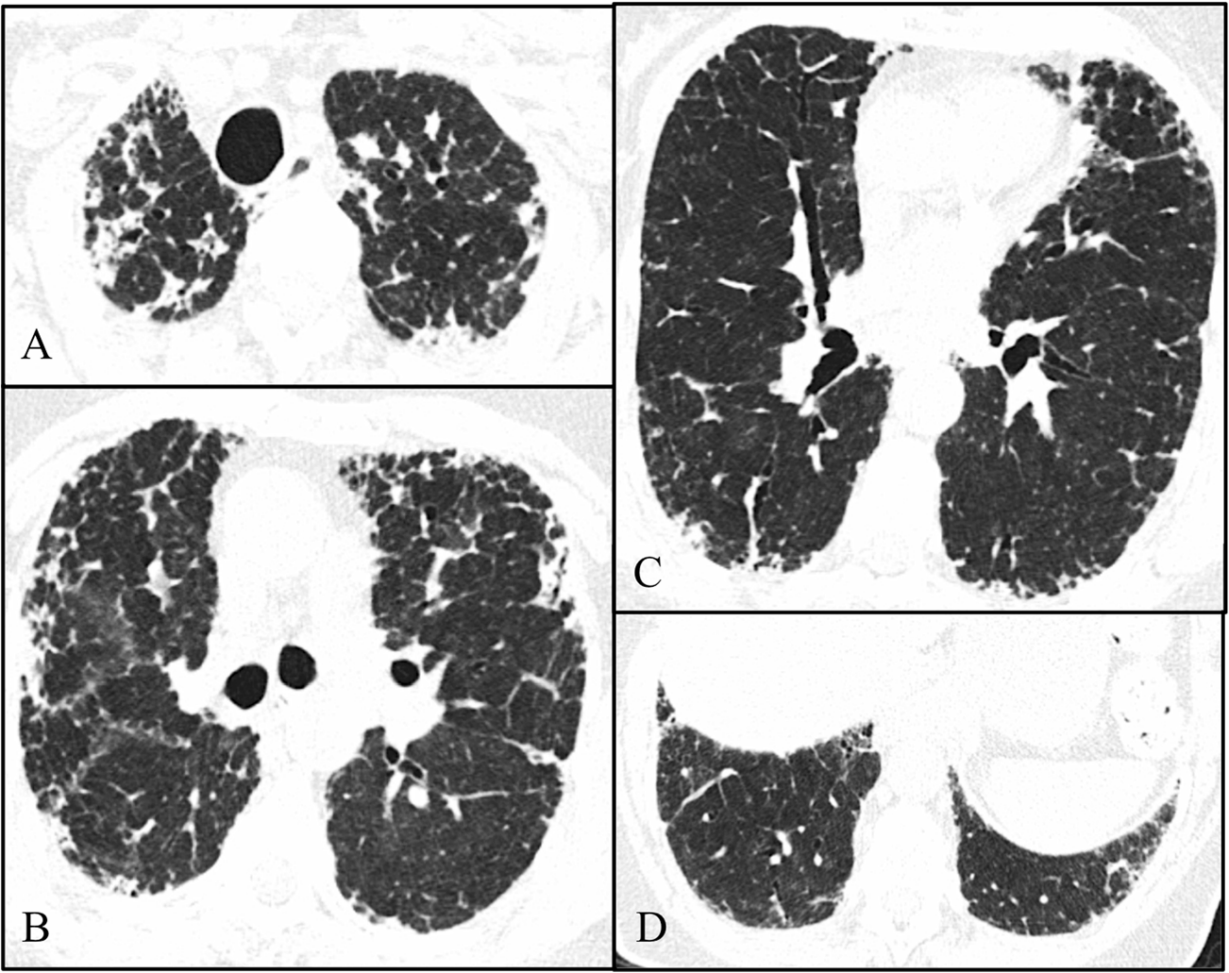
Axial high-resolution chest tomography (HRCT) images (a–c) and coronal reformatted images (d) of patient 1 show pleural and subpleural thickening with severe fibrotic changes in the marginal parenchyma with apical-caudal distribution. Some areas of traction honeycombing are also seen
